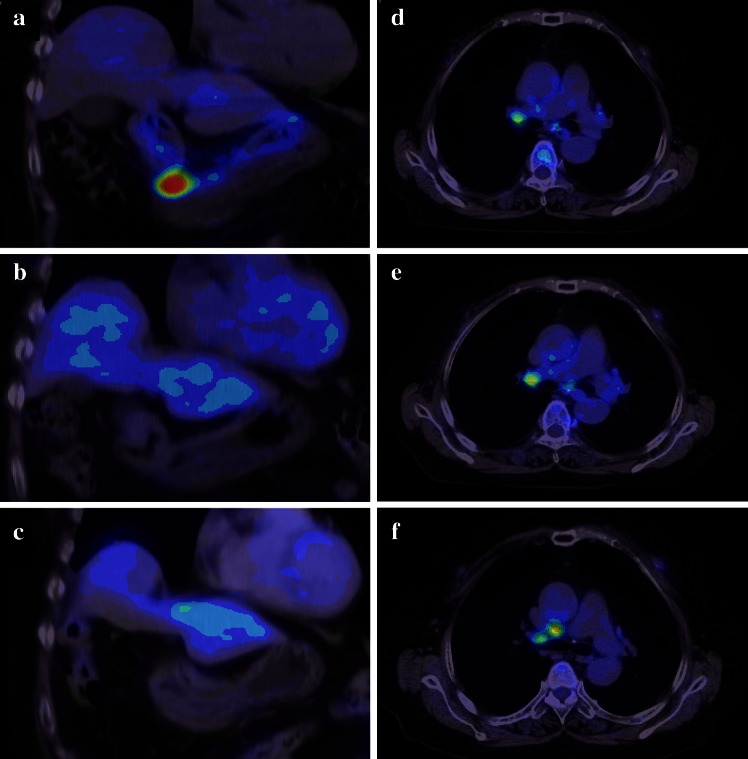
a–c Coronal PET-CT scan image of the stomach. d–f Axial PET-CT scan image of the mediastinum and chest wall. a Before cessation of MTX, increased FDG avidity was shown in the stomach wall. b Six months after cessation of MTX, no FDG avidity was seen in the stomach wall. c One year after chemotherapy, no FDG avidity was shown in the stomach wall. d Before cessation of MTX, slightly increased FDG avidity was visible in the mediastinum and left chest wall. e Six months after cessation of MTX, the slightly increased FDG avidity in the mediastinum and left chest wall remained unchanged. f One year after chemotherapy. Slightly increased FDG avidity in the mediastinum and left chest wall remained unchanged.
